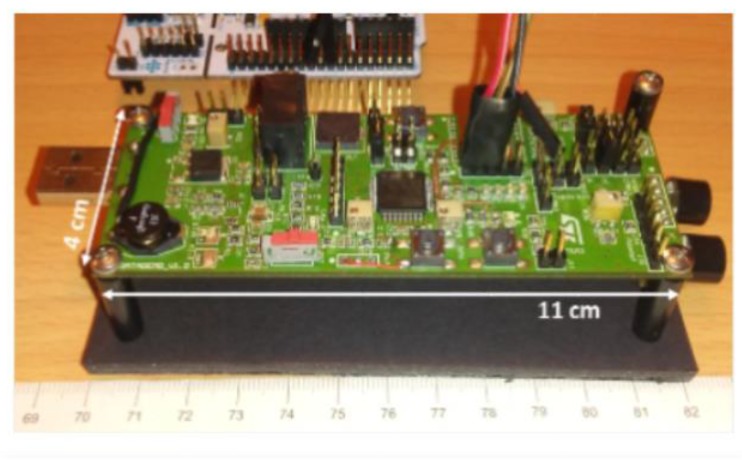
Ultrasound prototype module.
